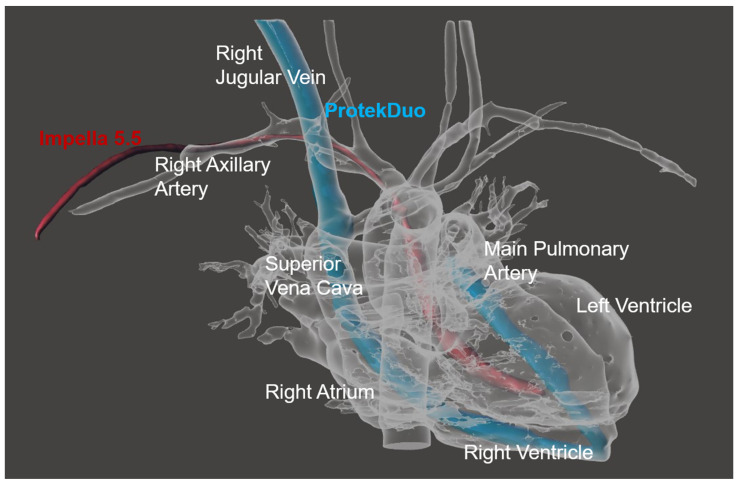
Three-dimensional reconstruction of a thoracic CT-scan of patient #3 while on hybrid-BiVAD support showing the Impella 5.5 pump (red) and the ProtekDuo cannula (blue).
